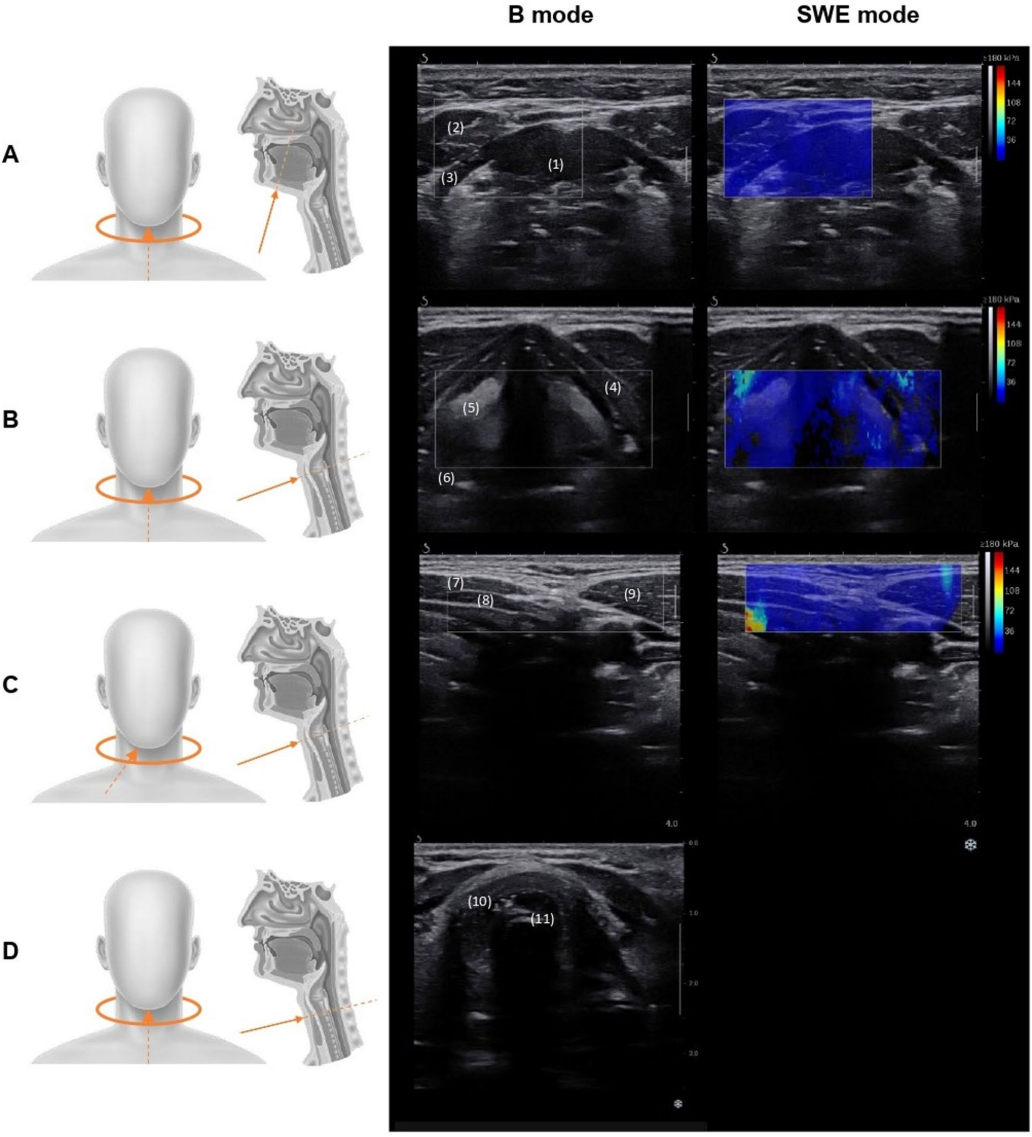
Ultrasound imaging plans showing probe position (left) and images obtained for each plan (right). Images of plans A, B and C were acquired in both B and SWE mode, images of plan D in B mode. Plan A: suprahyoid muscles plan – (1) geniohyoid muscle (2), anterior belly of the digastric muscles (3), mylohyoid muscle; Plan B: vocal cords plan – (4) thyroid cartilage (5), vocal folds (6), arytenoid cartilage; Plan C: infra-hyoid muscles lateral plan – (7) sternohyoid muscle (8), sternothyroid muscle (9), sternocleido-occipito-mastoid muscle; Plan D: cricoid cartilage plan – (10) cricoid cartilage (11), intubation tube artefacts
